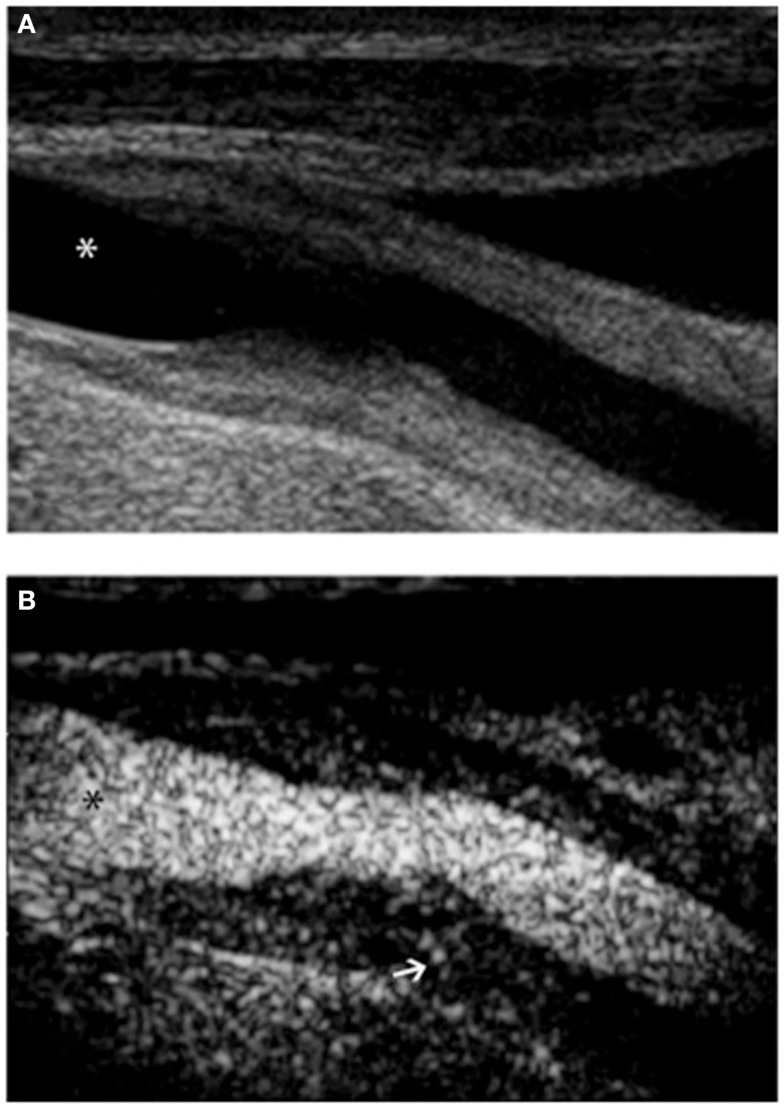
Ultrasound examination of right proximal common carotid artery of a patient affected by Takayasu arteritis. B-mode ultrasound (A) shows long, smooth concentric thickening of the arterial wall. Contrast-enhanced ultrasound (B) using Optison (GE Healthcare, Little Chalfont, UK), a contrast media made up of human albumin microbubbles filled with perflutren, improves definition of the lesion border. Extensive enhancement can be seen within the vessel wall (arrow). In both panels, asterisk marks the vessel lumen.
